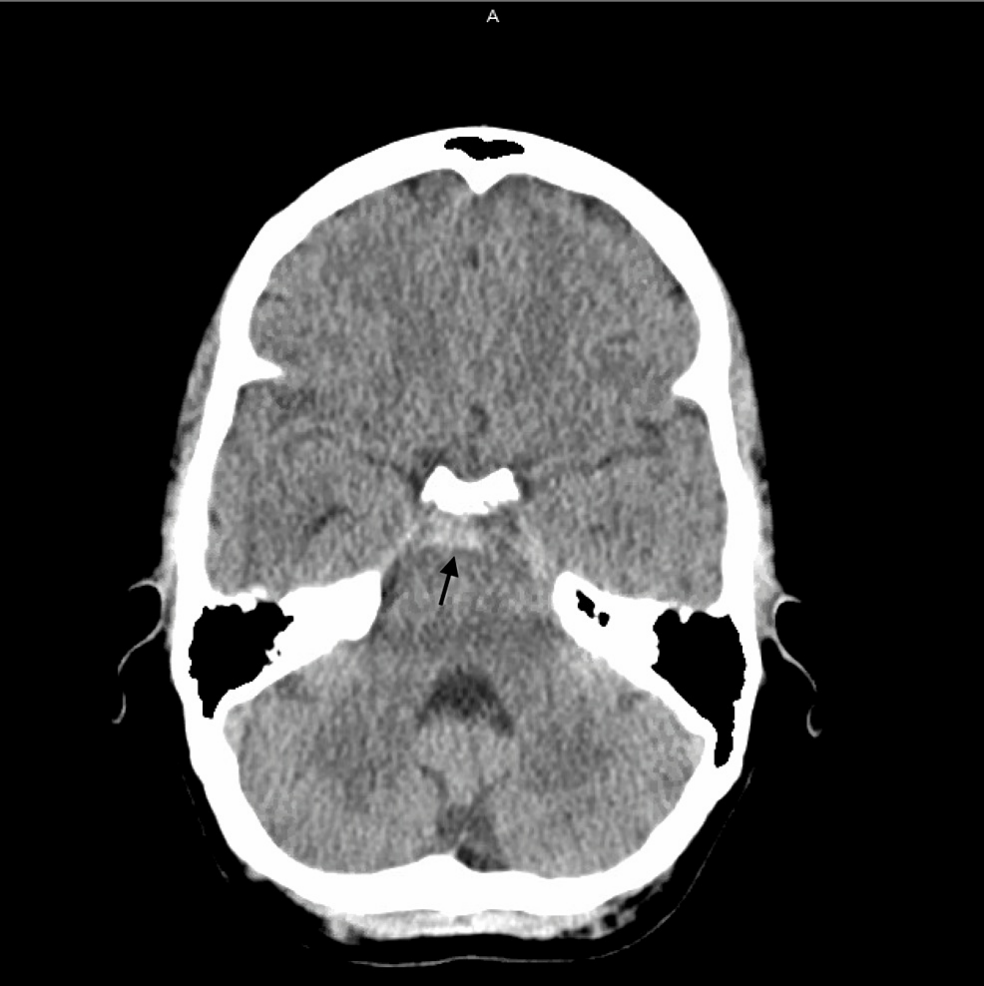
Head CT findings compatible with acute subarachnoid hemorrhage involving portions of the suprasellar, interpeduncular, and prepontine cisterns.
